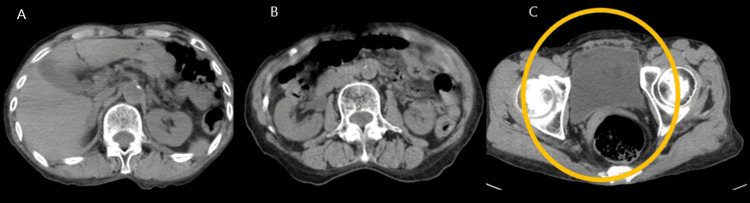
Noncontrast CT of the abdomen. (A) CT image showing no signs of adrenal abnormality. (B) Ureteral dilation is observed on the CT image; however, there are no visible ureteral stones. (C) CT image showing bladder distention with urinary retention. The yellow circle indicates urine retention in the bladder. CT: computed tomography
